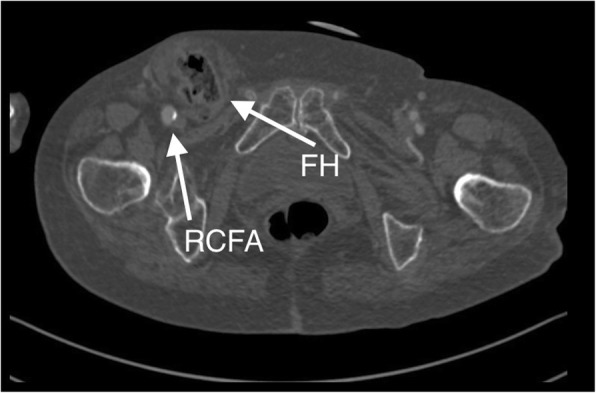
Computed tomography angiography (CTA) transverse cross-sectional image. Right common femoral artery (RCFA), femoral hernia (FH) sack
